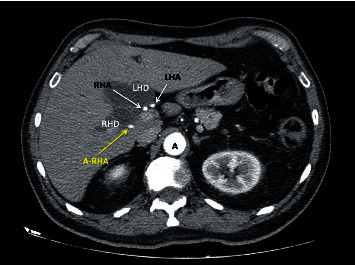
CT images of a patient with a periampullary tumor and a dilated biliary tree. The dilated left (LHD) and right hepatic ducts (RHD) are seen at the liver hilum. Corresponding to this, the proper hepatic artery has divided normally into a left hepatic artery (LHA) and right hepatic artery (RHA) at the hilum. This patient also has an accessory right hepatic artery (A-RHA) as a type 6 variant.
